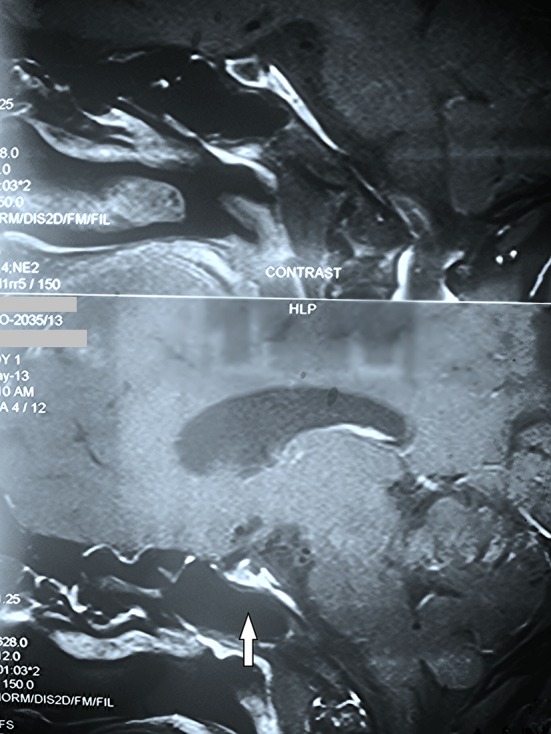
Sagittal view MRI of the patient revealing empty sella (arrow).
